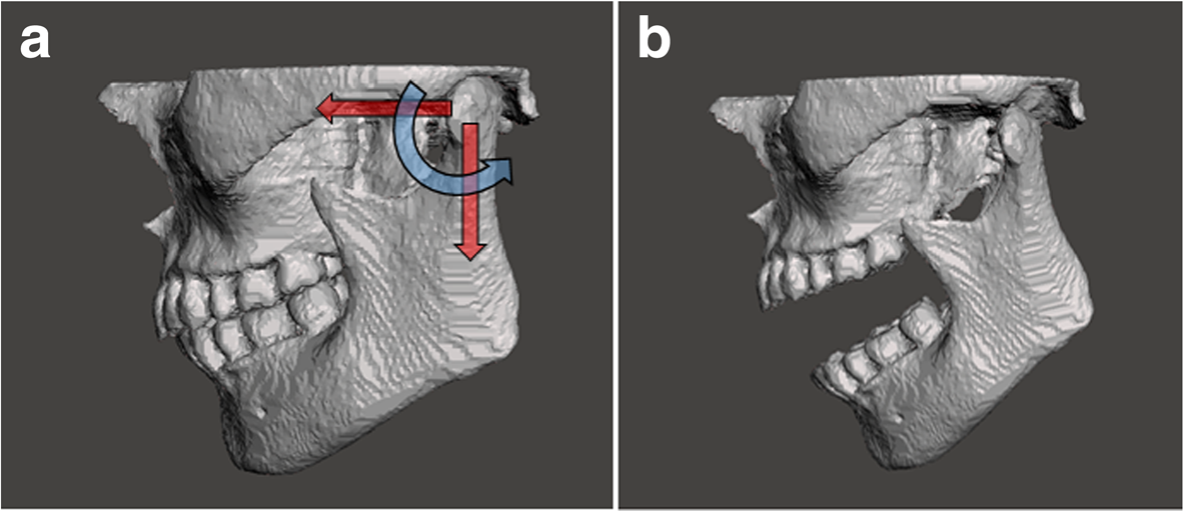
a) 3D CAD model obtained from the CT image dataset. The mandible was rotated and translated anteriorly and inferiorly to produce b) the jaw position with the desired incisal opening
